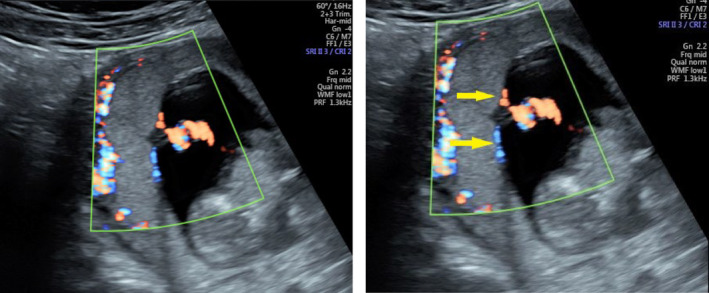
Power Doppler demonstrates the branching of vessels (arrows) at the PCI site. PCI, placental cord insertion.
